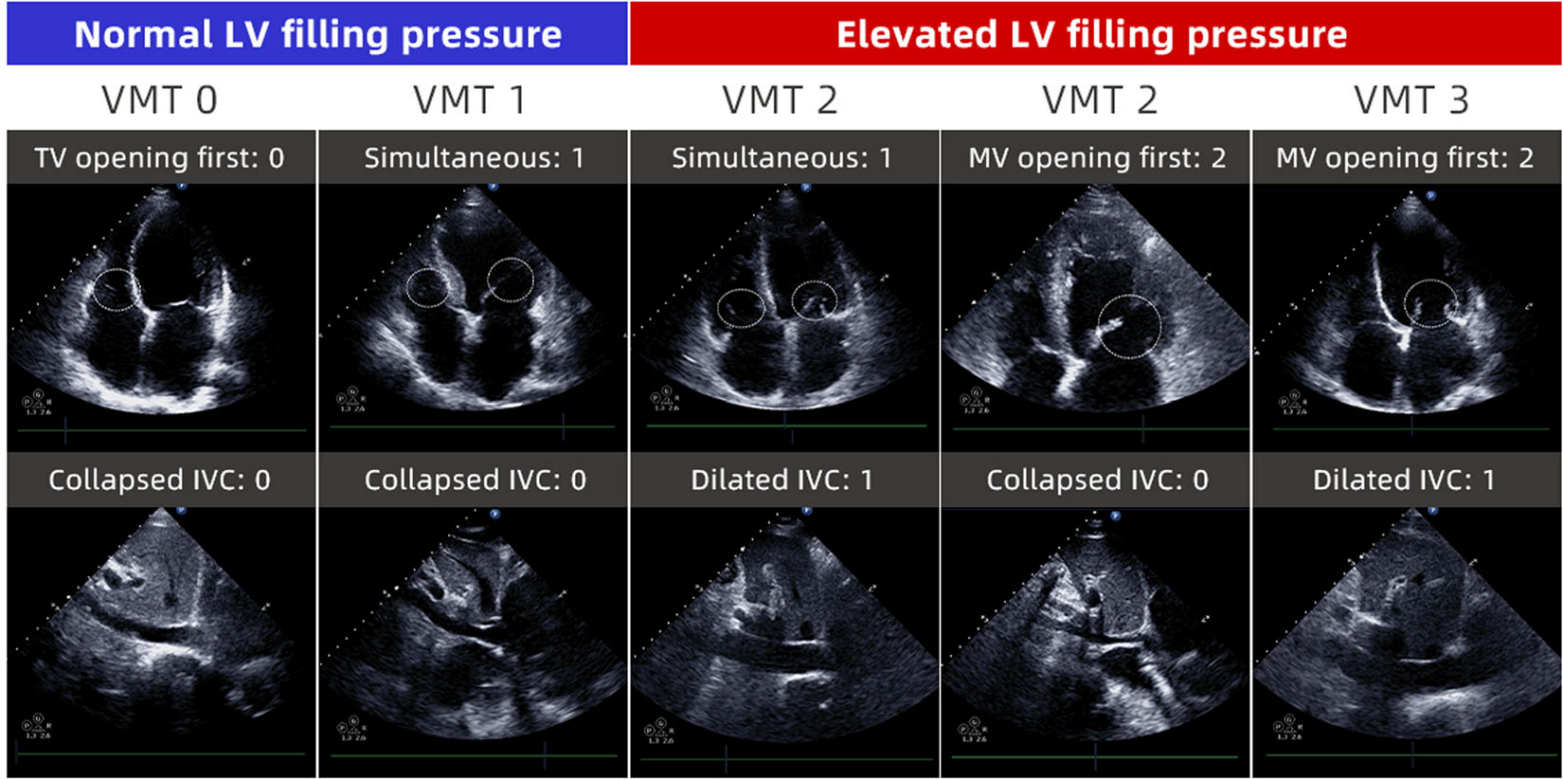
The echocardiographic images of different mitral valve and tricuspid valve opening (VMT) scores.
